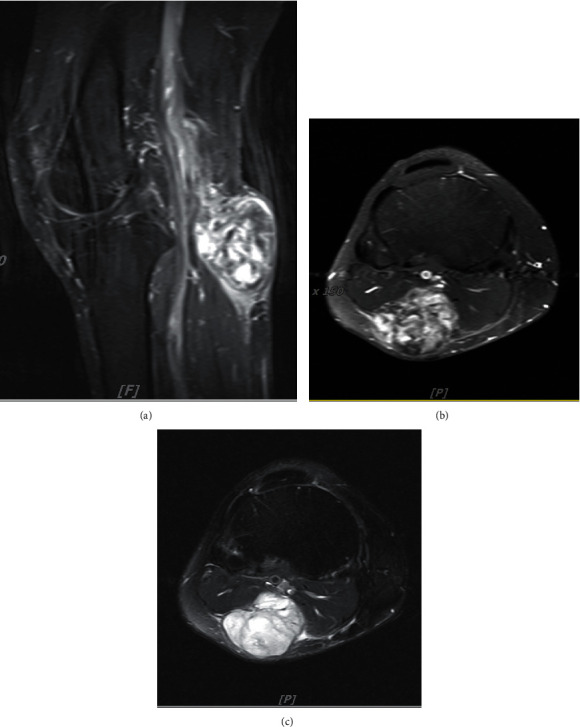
Magnetic resonance imaging. (a) Sagittal view with gadolinium contrast. (b) Axial view with gadolinium contrast. (c) Axial T2-weighted image.
